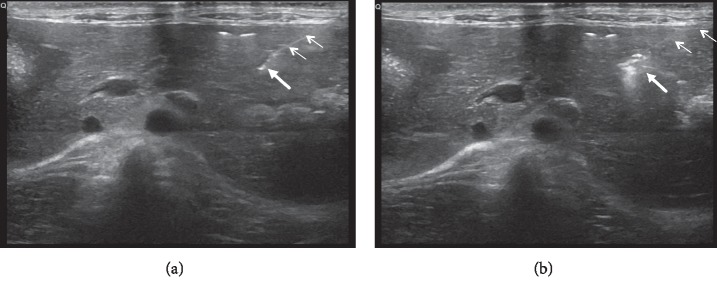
Ultrasound image of the established model by interventional method. Ultrasound-guided percutaneous puncture of 18G PTC needle into the liver, and the small arrows mark the needle path and the thick arrow marks the tumor tissue of the target area (a). PTC needle tip of the high echo mass is pushed into the liver tumor tissue fragments and is accompanied by a small amount of gas, where the small arrows mark the needle path and the thick arrow marks the tumor tissue (b).
